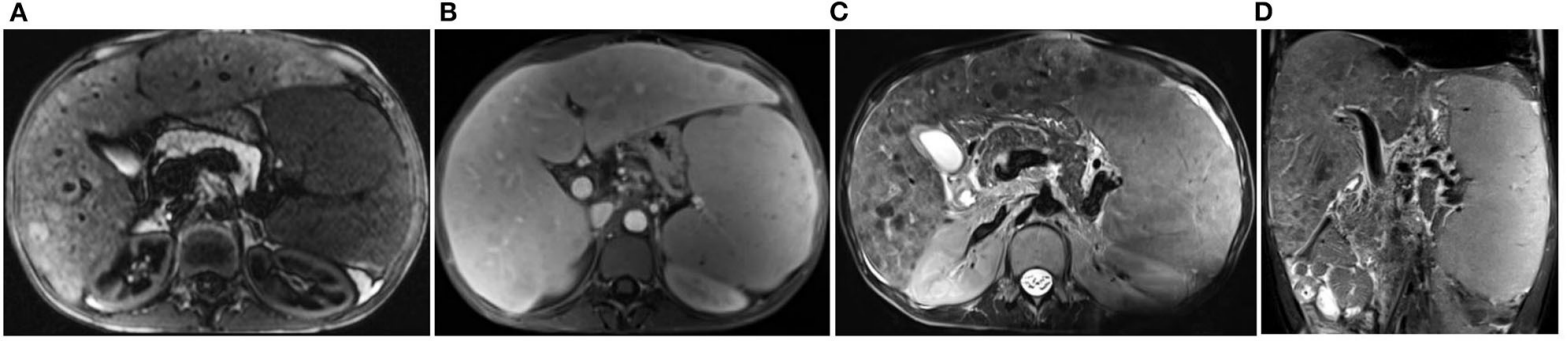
Magnetic resonance imaging of the abdominal cavity. Markedly enlarged spleen (19.1 × 8.2 cm) with irregular, nodular reconstruction, with hypointense foci in T1 and T2 dependent images, 5–60 mm large, modeling on vessels, and signal loss flow void foci in the central part of the spleen. The liver enlarged, the right lobe 18 cm, left lobe 14 cm, both lobes showing irregular nodular reconstruction, in T1 image hyperintense, in T2 image hypointense. All images show different phases of one MRI examination in the patient aged 17. (A) Transverse view, T1-weighted gradient-echo HASTE (Turbo spin-echo) sequence, oppose-phase imaging. (B) Transverse view, 3D starVIBE T1-weighted gradient-echo sequence FS (fat-suppressed), contrast protocol. (C) Transverse view, T2 image with SPC pulse sequence. (D) Coronal view, T2 image HASTE FS sequence.
